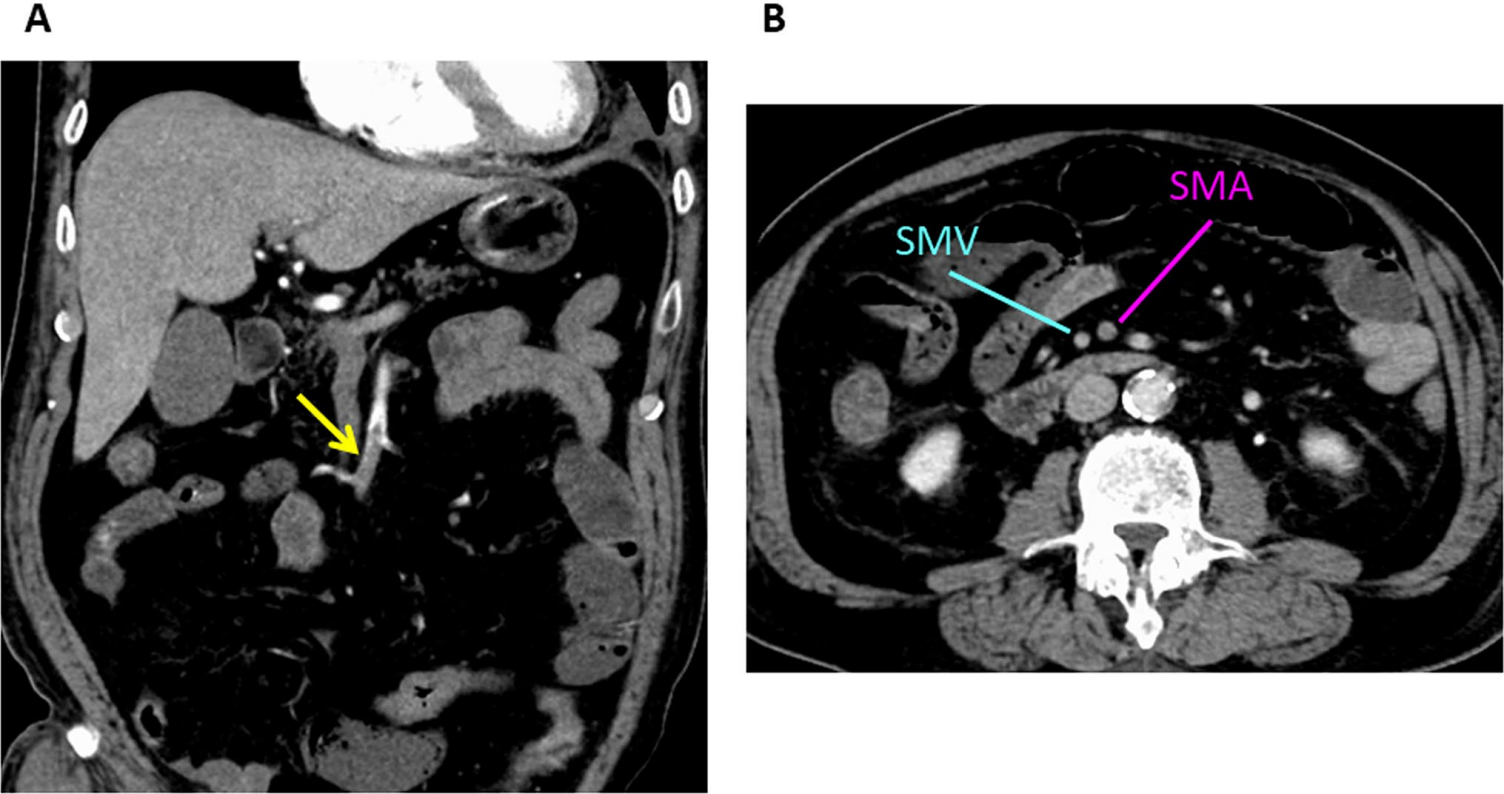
Superior mesenteric artery embolism in a 66-year-old man immediately after surgery for left lung cancer. A Coronal contrast-enhanced CT image (arterial phase) showing an occluding embolus (arrow) in the superior mesenteric artery. B On the contrast-enhanced CT image (venous phase), the diameter of the superior mesenteric vein (SMV) is smaller than that of the superior mesenteric artery (SMA)
